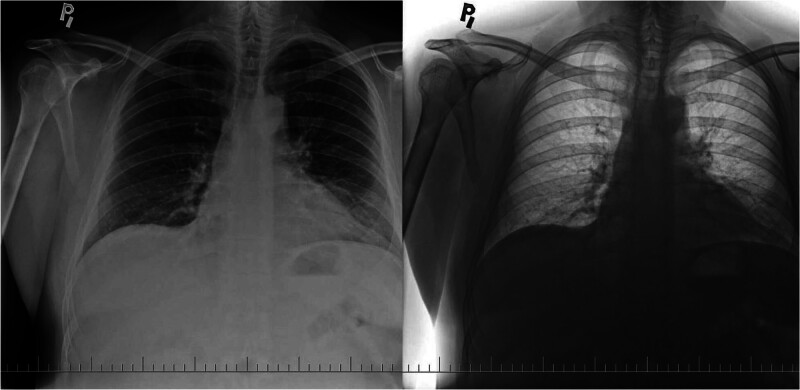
Chest X-ray in Posterior-Anterior projection of lungs. April 2018 local hospital’s surgical department.
